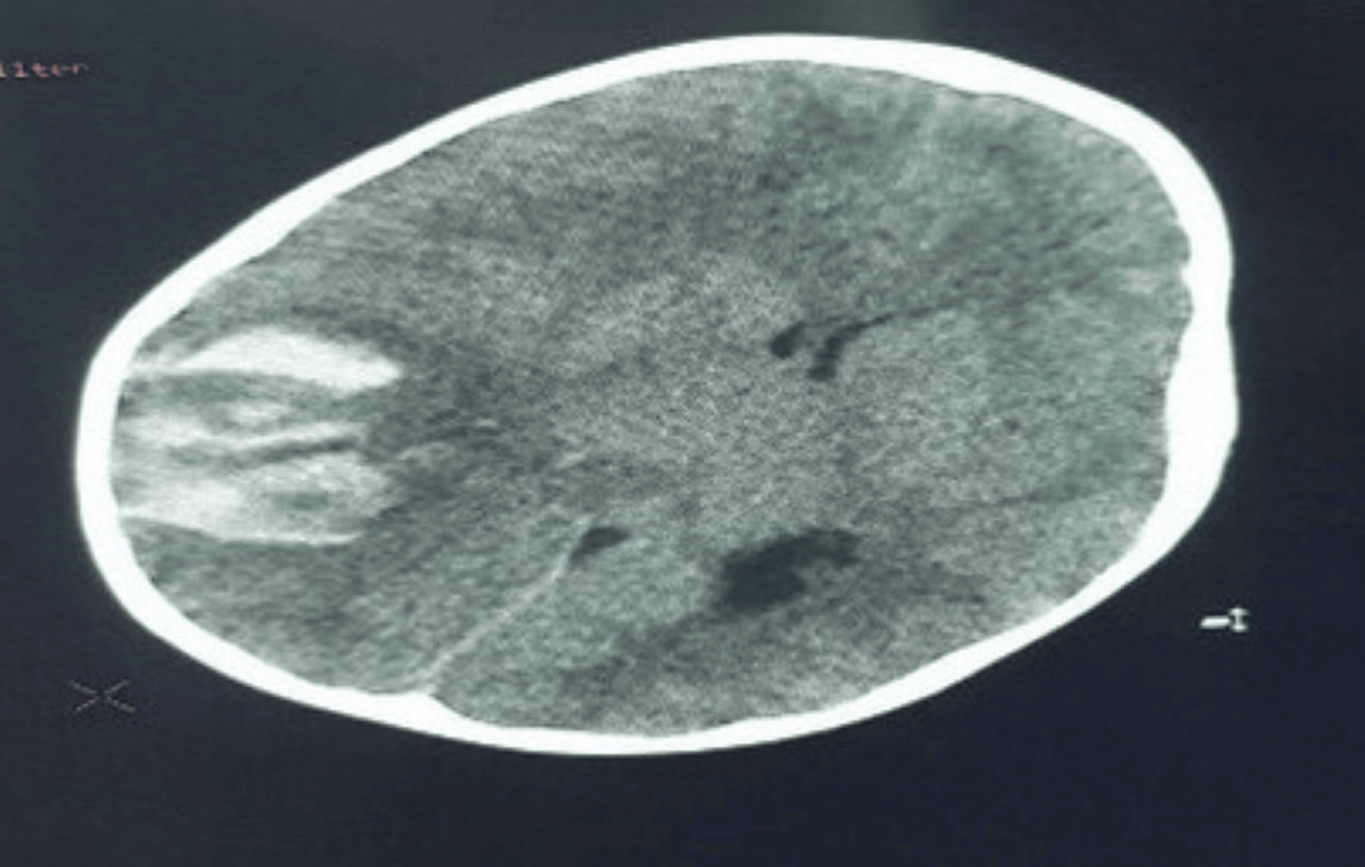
Non-contrast Head CT Showing Left-Sided Intracerebral Hemorrhage with a Midline Shift
